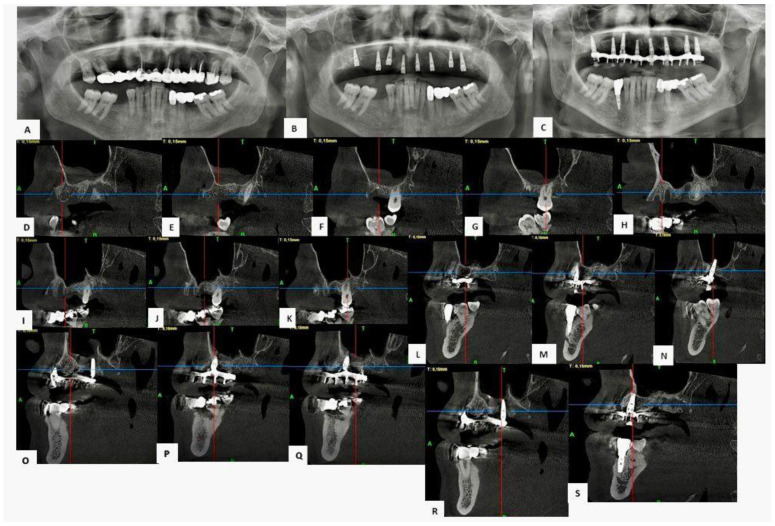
(A) Pre-operative OPT. (B) OPT check after placement of endosseous implants. (C) OPT check after 84 months. (D–K) Pre-operative CBCT sections of areas 1.4, 1.5, 1.6, 1.7, 2.4, 2.5, 2.6 and 2.7. (L–S) CBCT sections after 84 months of areas 1.4, 1.5, 1.6, 1.7, 2.4, 2.5, 2.6 and 2.7.
